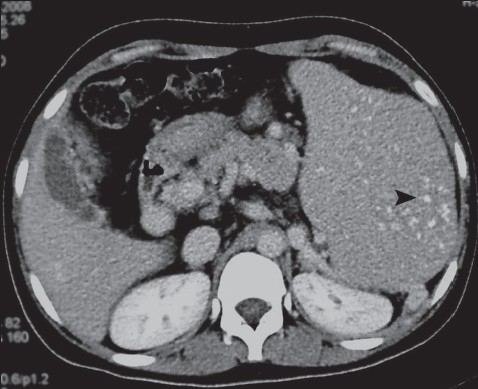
Non-enhanced abdominal CT scan showing massive splenomegaly with multiple tiny, discrete, hyperdense lesions within the spleen (black arrow).
